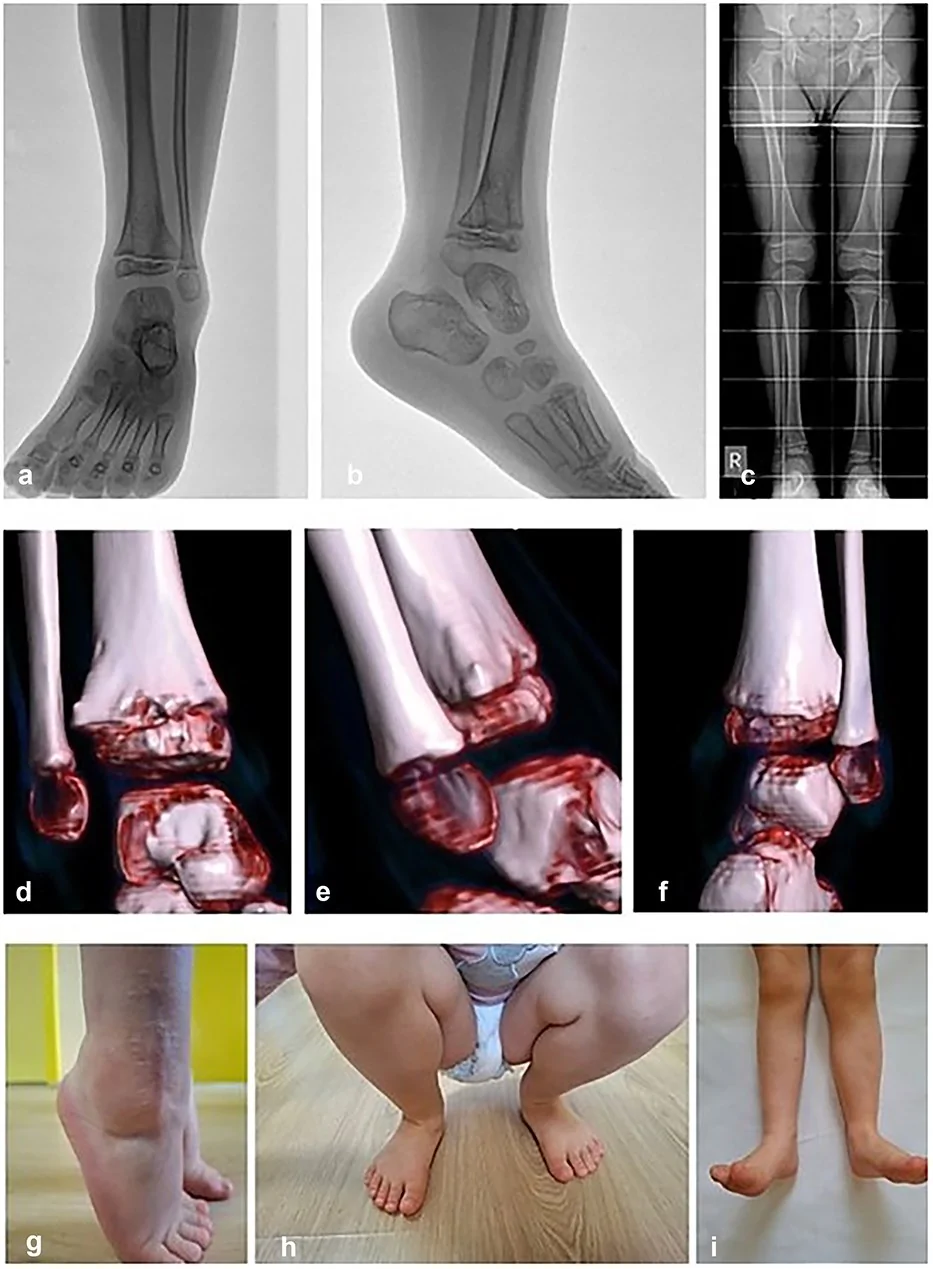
(a–c) Plain radiographs obtained 1 year and 6 months postoperatively demonstrating advanced consolidation of the lesion; the distal tibial physis shows preserved growth, and the lower limbs are of equal length; (d–f) three-dimensional CT scans of the ankle and foot 18 months after surgery; (g–i) clinical photographs demonstrating full ROM of the ankle and foot.
